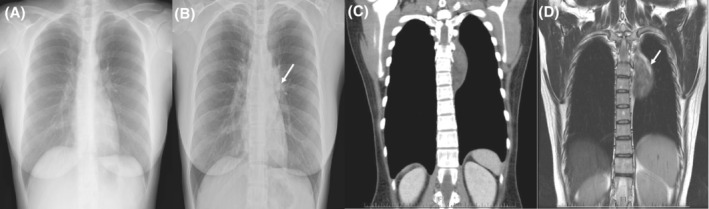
Chest x‐ray. (A) No mediastinum mass noted 4 years prior to visit. (B) A faint bulging mass (white arrow) was noted at the posterior mediastinum, left paraspinal region. (C) Computed tomography of the intrathoracic tumour: heterogeneous density with contrast‐enhanced fat‐containing lesion at the left paraspinal region. (D) Magnetic resonance imaging of the intrathoracic tumour, T2‐weighted image (sagittal view): heterogeneous intensity fat‐containing lesion at the paraspinal region (arrow)
